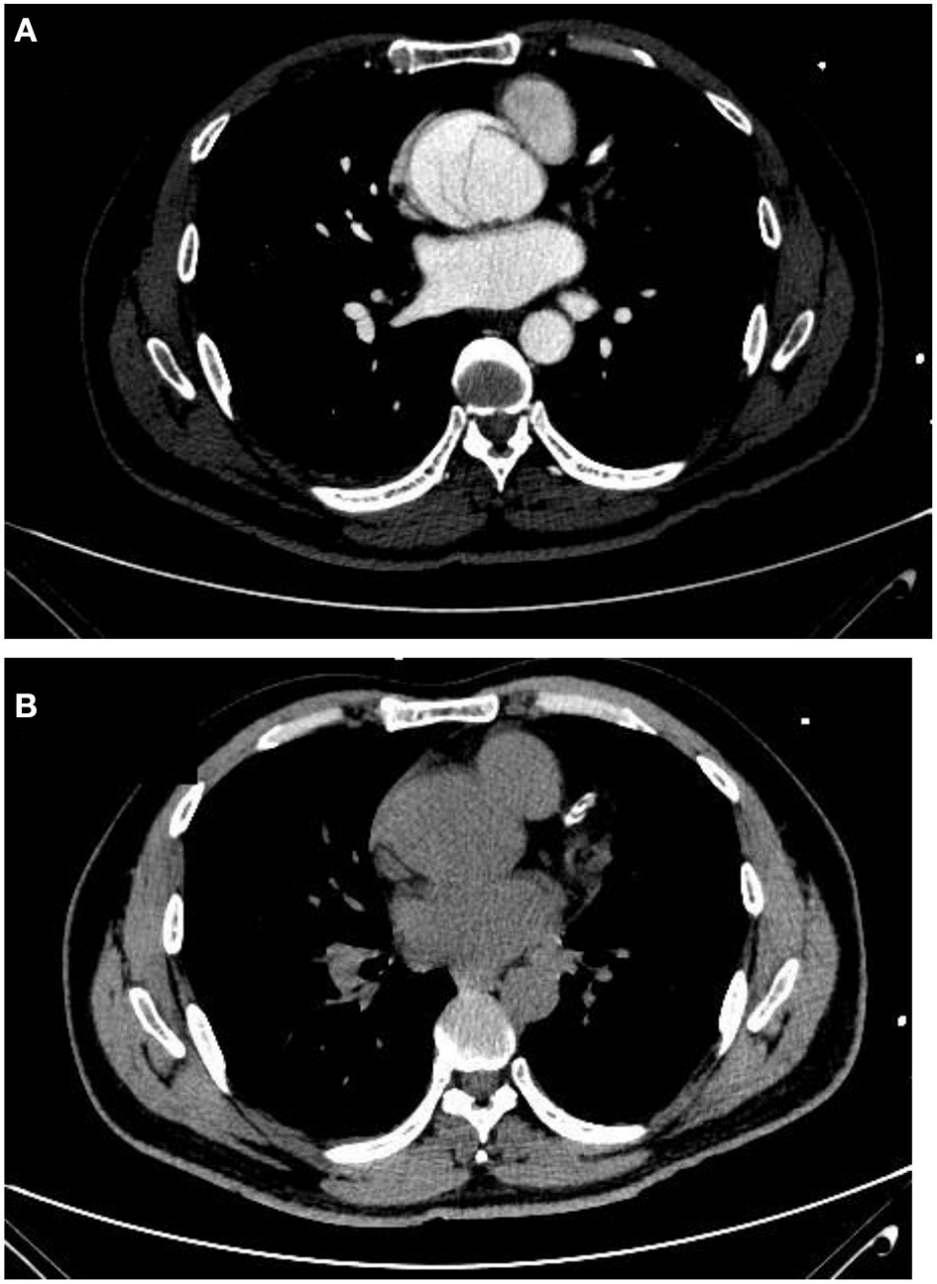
(A) MDCT image of the LAD in an enhancement scan. (B) MDCT image of the LAD in a plain scan. LAD, left anterior descending coronary artery; MDCT, multidetector computed tomography.
